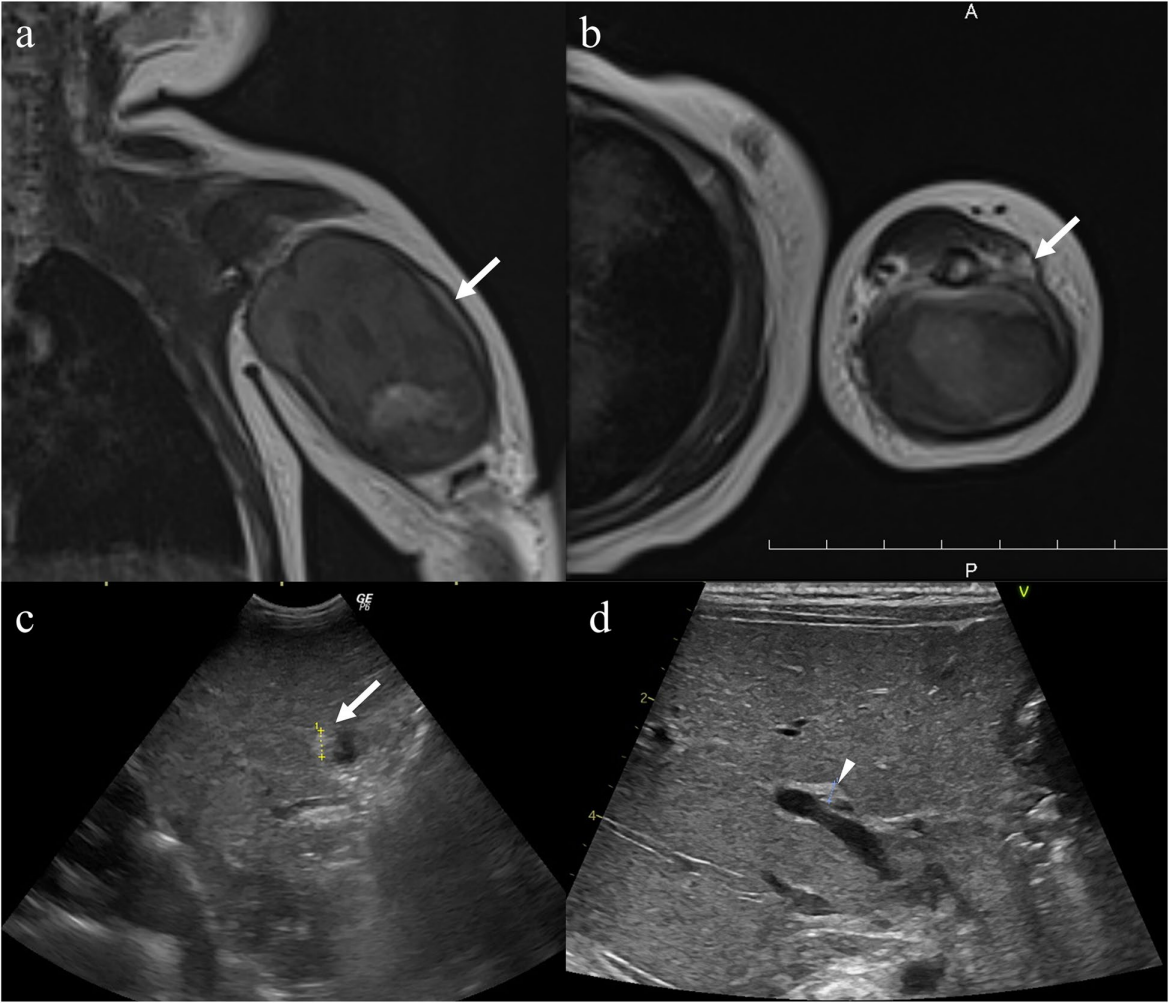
Coronal (a) and axial (b) magnetic resonance images of the left upper arm. The arrows indicate a hematoma in the triceps brachii. No changes in signal intensity were observed in the subcutaneous tissue. The radial nerve was not clearly visualized. c and d Ultrasonographic images of the abdomen. The arrow in c indicates the gallbladder (diameter, 7.5 mm). The arrow head in d indicates the triangular cord sign anterior to the bifurcation of the portal vein
